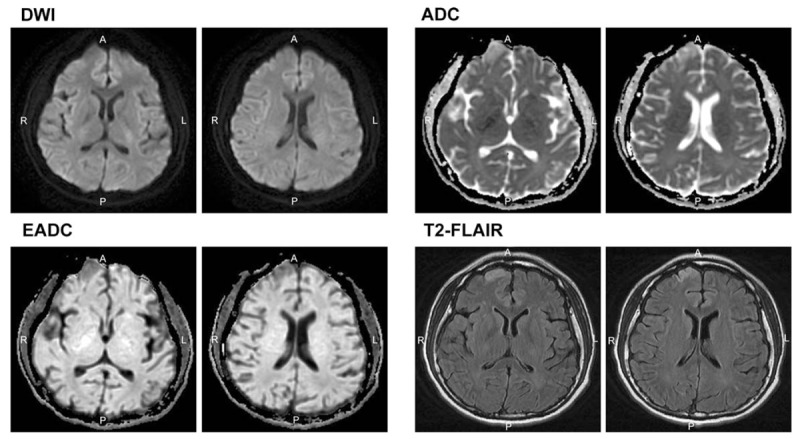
Brain magnetic resonance imaging (MRI) 2 months later showed high signal on T2-weighted (T2W) and fluid-attenuated inversion recovery (T2-FLAIR) images and low-signal intensity on T1-weighted (T1W) images in the right frontal lobe, suggesting encephalomalacia.
